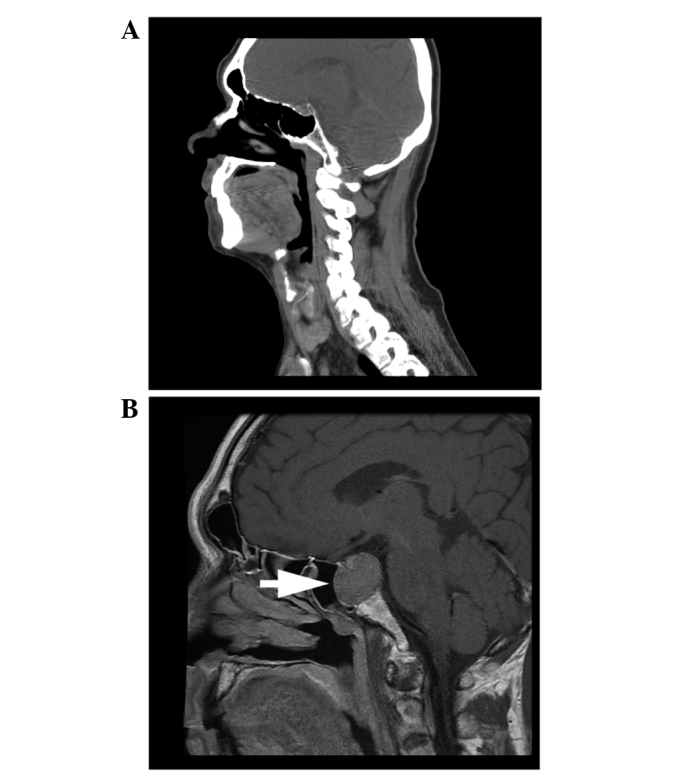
Sagittal view on T2-weighted magnetic resonance imaging at (A) two and (B) four months after completing treatment for hypopharyngeal carcinoma. A well-defined tumour in the pituitary fossa was revealed in subsequent imaging (arrow).
